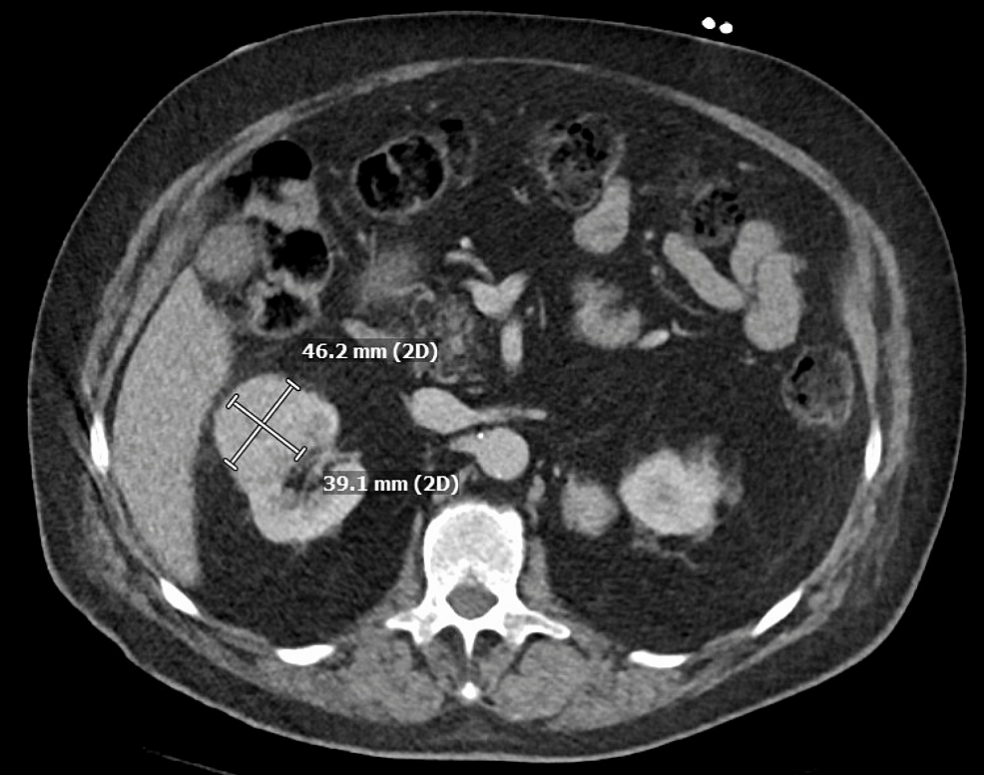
CT abdomen pelvis demonstrated a 4.6 x 3.9 x 4.3 cm right upper renal lesion. CT: computed tomography
